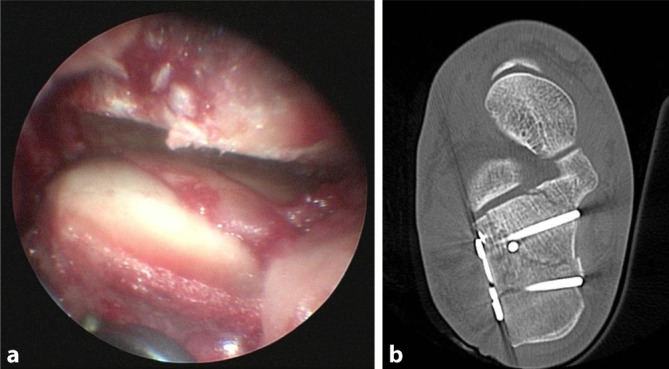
a Frakturoskopie, b postoperativer paraaxialer CT-Schnitt nach Versorgung einer Calcaneus-Fraktur Typ 2AC nach Sanders
